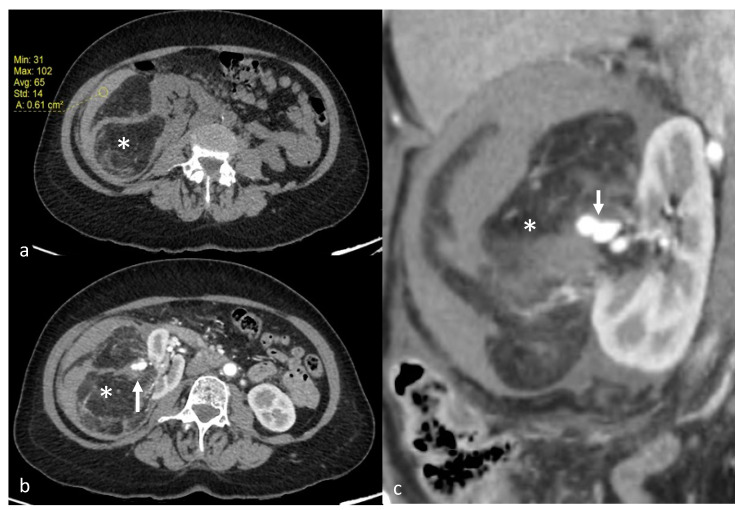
A 70-year-old patient with right AML (*), Wunderlich Syndrome and aneurysm (white arrow). (a) Yellow ROI showing retroperitoneal hemorrhage (65 UH) on axial computed tomography without injection; (b) Axial computed tomography with injection and arterial acquisition; (c) Coronal computed tomography with injection and arterial acquisition.
